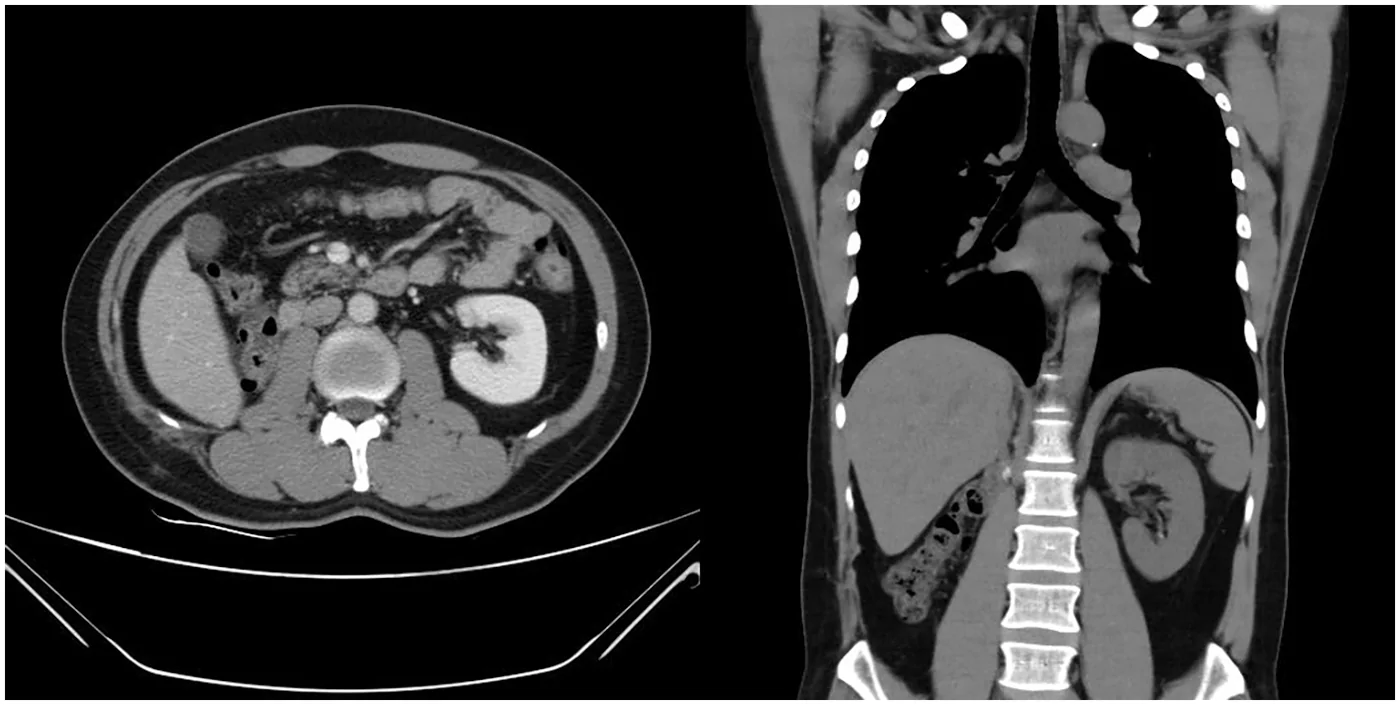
A follow-up enhanced CT scan of the chest, abdomen, and pelvis one year after comprehensive postoperative treatment showed no active tumors.
